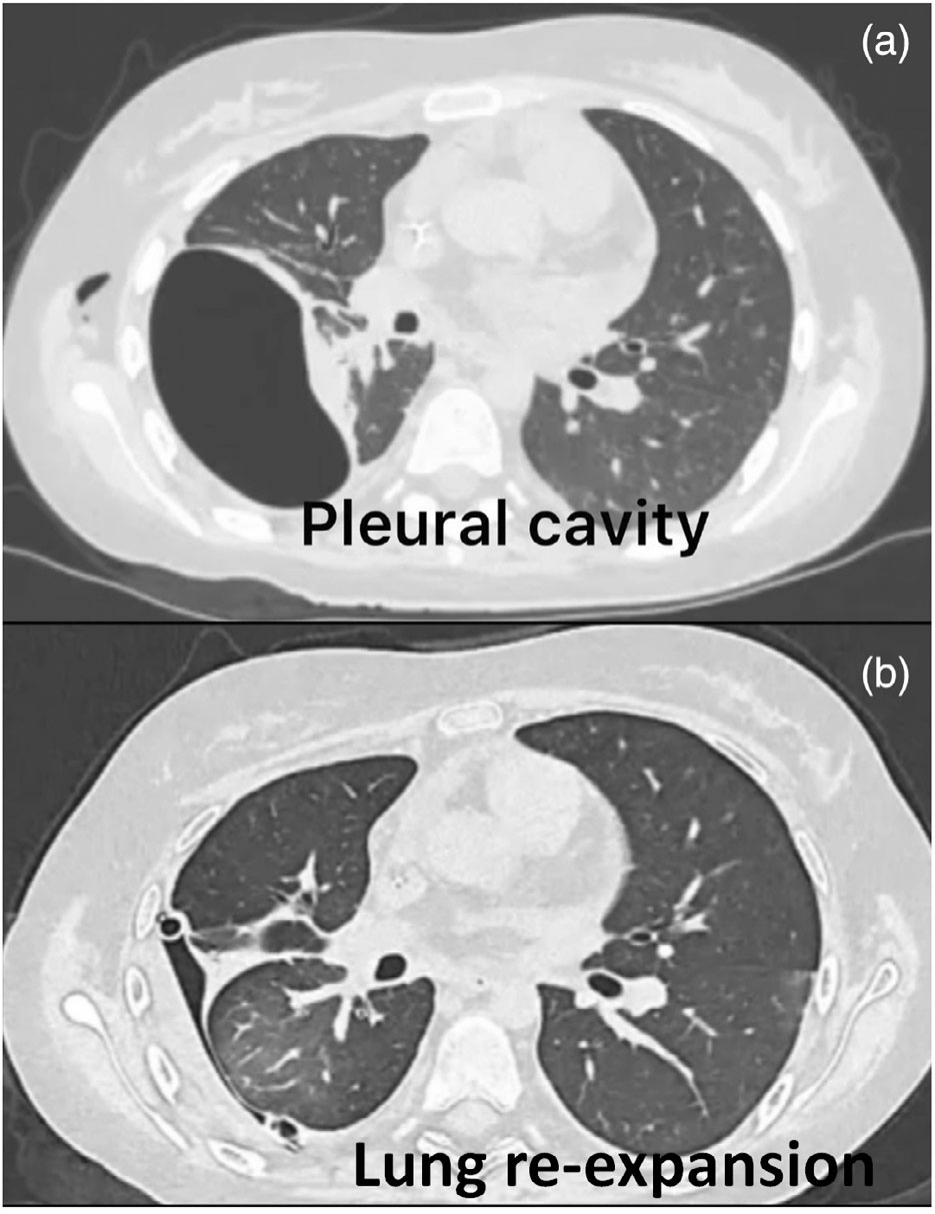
(a) Chest computed tomography (CT) scan revealed a loculated pneumothorax within the right upper lobe due to the presence of persistent air leaks. (b) Chest CT scan performed 2 days after treatment showed re‐expansion of the lung with the resolution of air leaks.
